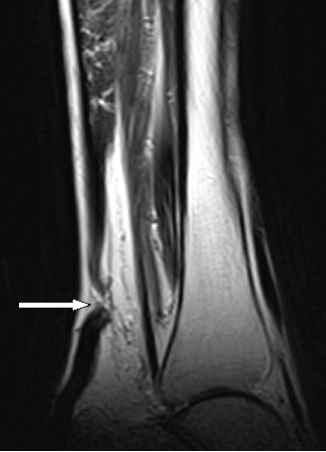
Sagittal view MRI scans of the patient's left ankle showing rupture of the Achilles tendon.
